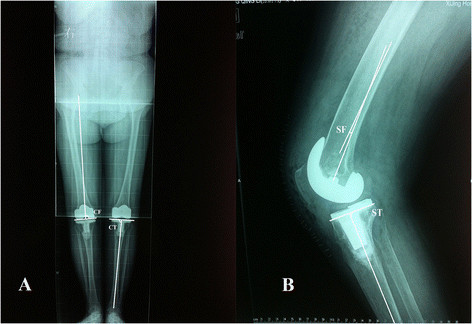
Measurement of various angles on anteroposterior (A) and lateral (B) radiographs after total knee arthroplasty. The component alignments were evaluated by measuring four modified angles including the coronal femoral angle (*CF*), coronal tibial angle (*CT*), sagittal femoral angle (*SF*) and sagittal tibial angle (*ST*).
